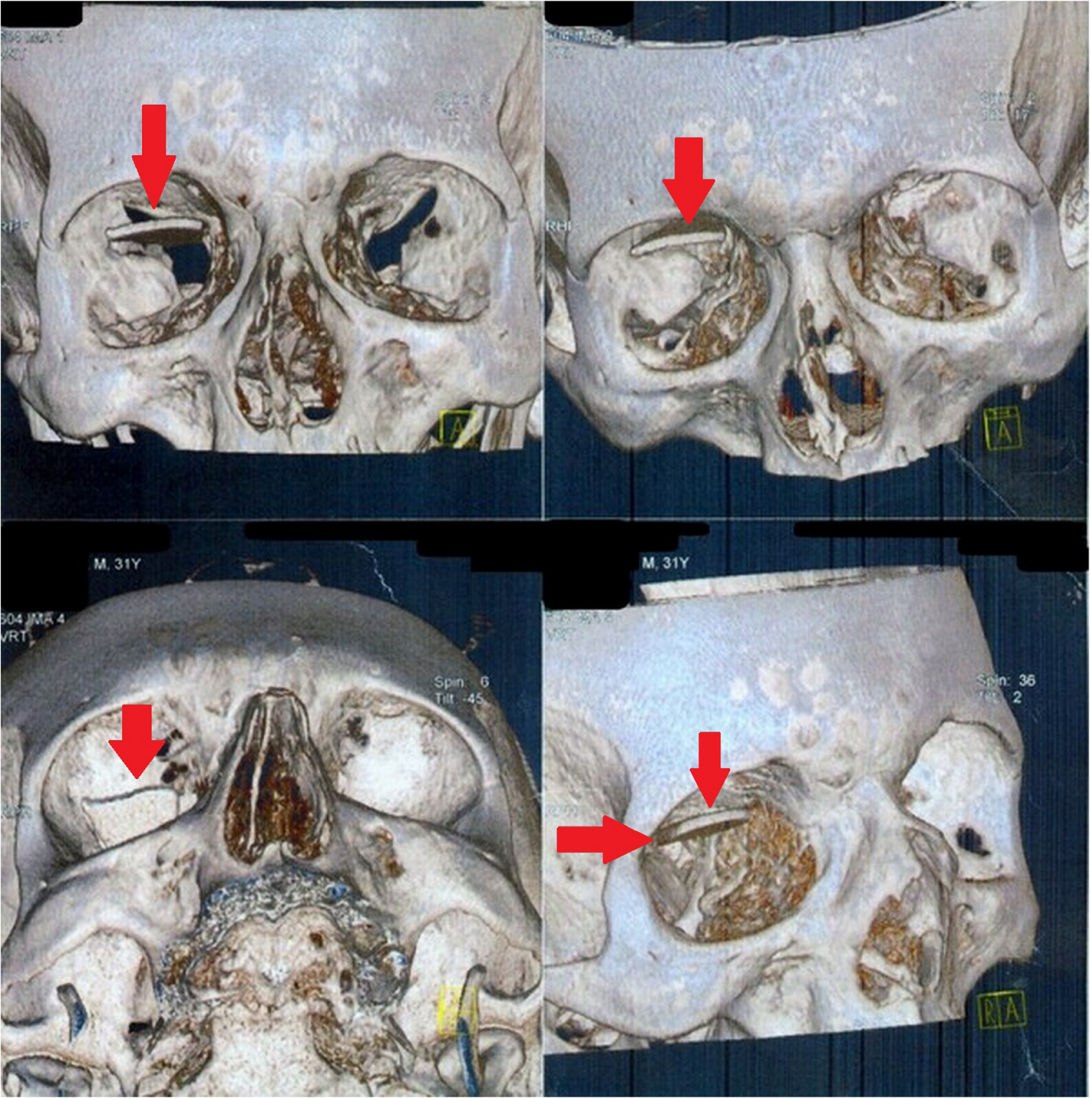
3D-Reconstructed Head CT (the object is seen in right orbital fossa)
